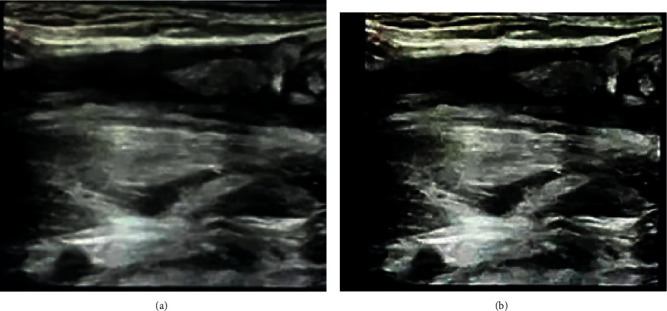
Comparison of the effect of scapula ultrasound before and after the artificial intelligence enhancement. (a) Unenhanced ultrasound image of the scapula. (b) Enhanced ultrasound image of the scapula.
